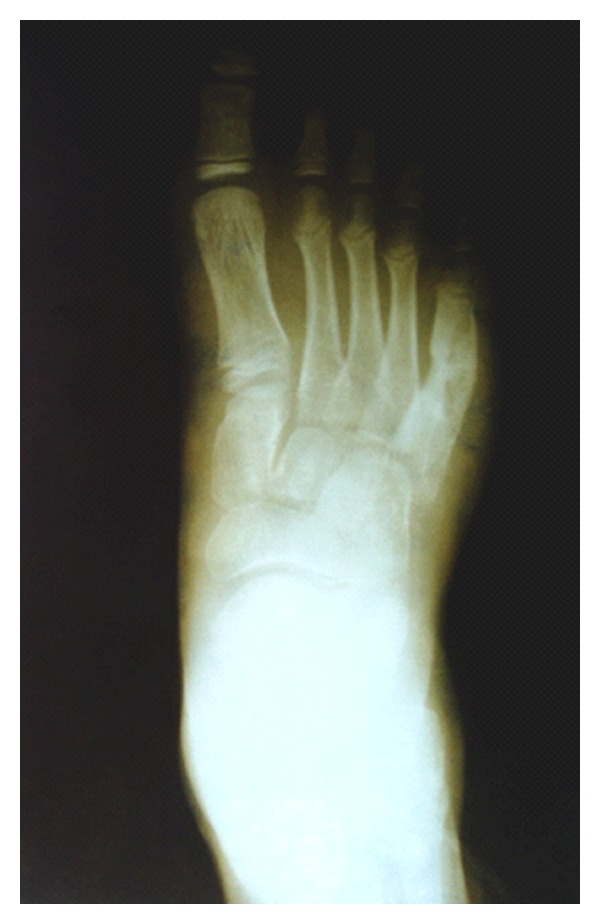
A radiograph of the right foot showed an eccentric lytic lesion of the cortex and the medullary space in the diaphysis of the fifth metatarsal with sclerotic margins.
